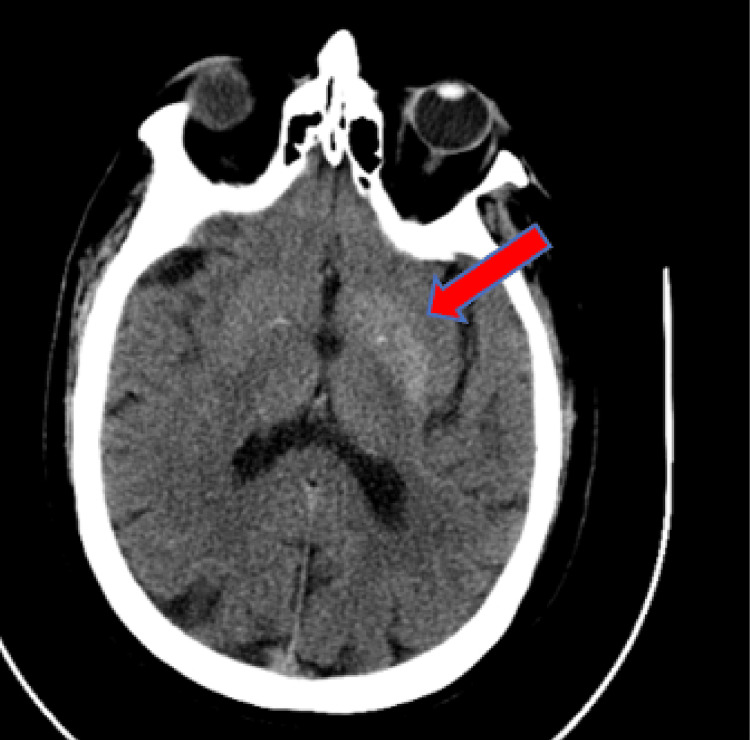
Head CT without contrast (subsequent slice) Hypodensity in the left putamen and globus pallidus (arrow) CT: computed tomography
